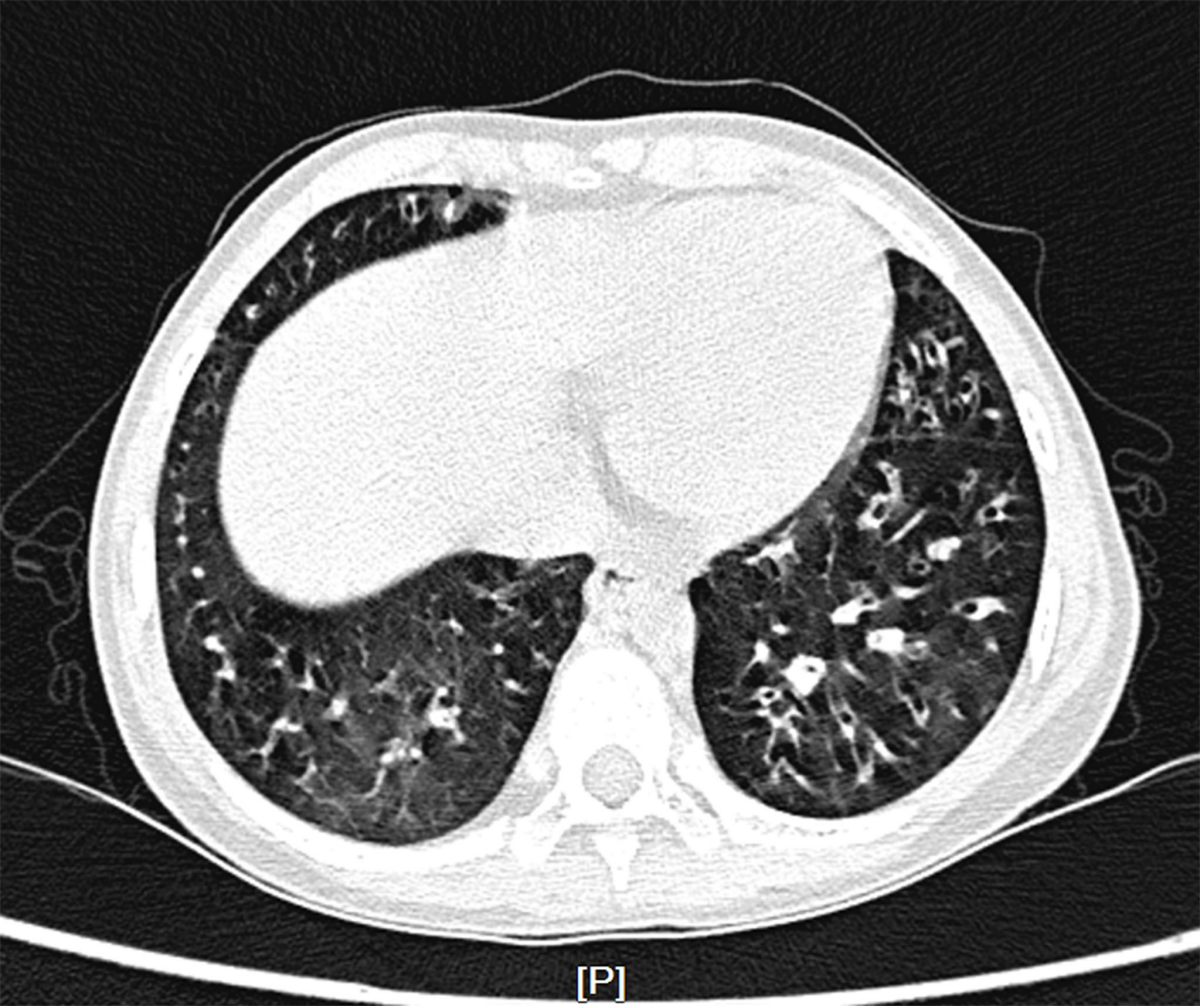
Chest computed tomography scan. Multiple bronchiectasis were randomly distributed in the bilateral lobe.
